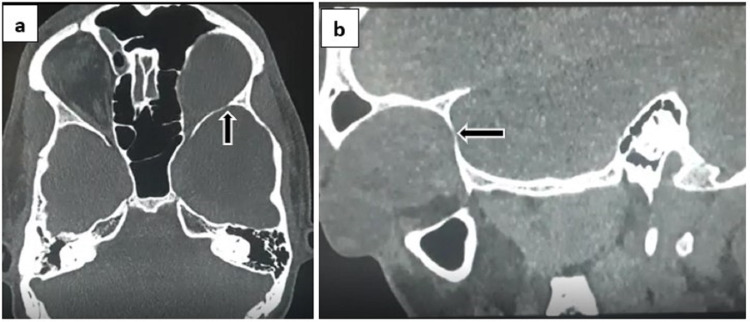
(a) CT axial section and (b) reformatted sagittal oblique view showing scalloping of left sphenoid wing with a small bony defect (black arrow) communicating intraorbital and intracranial cystic lesions.
